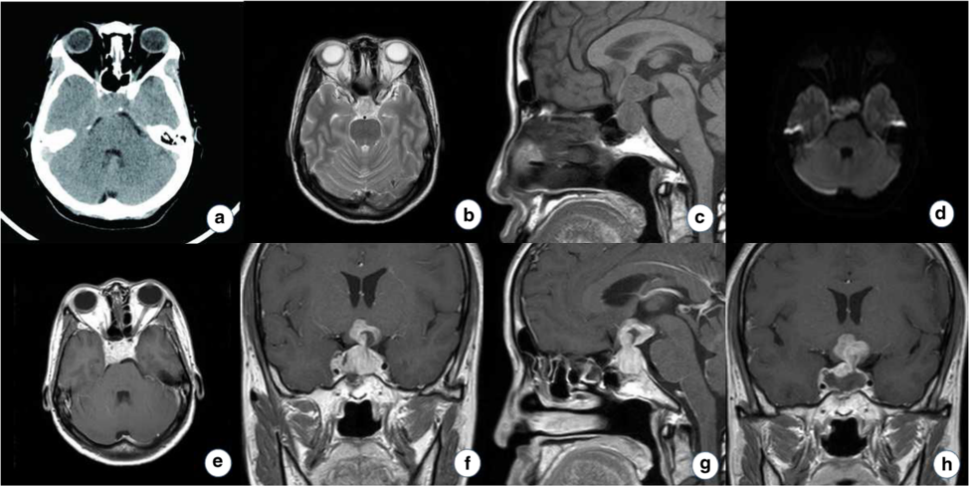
The neuroimaging results of the first patient. a CT scans demonstrated an oval high-density lesion in the sellar area and bone destruction. b T2-weighted MRI showed the lesion was slight hyperintense. c T1-weighted sagittal MRI showed the lesion was isointense. d DWI-MRI showed the lesion was iso- or hyperintensity. e–g The solid part heterogeneously enhanced after contrast. h. Postoperative MRI scans (3 days later) showed a gross removal of the tumor
